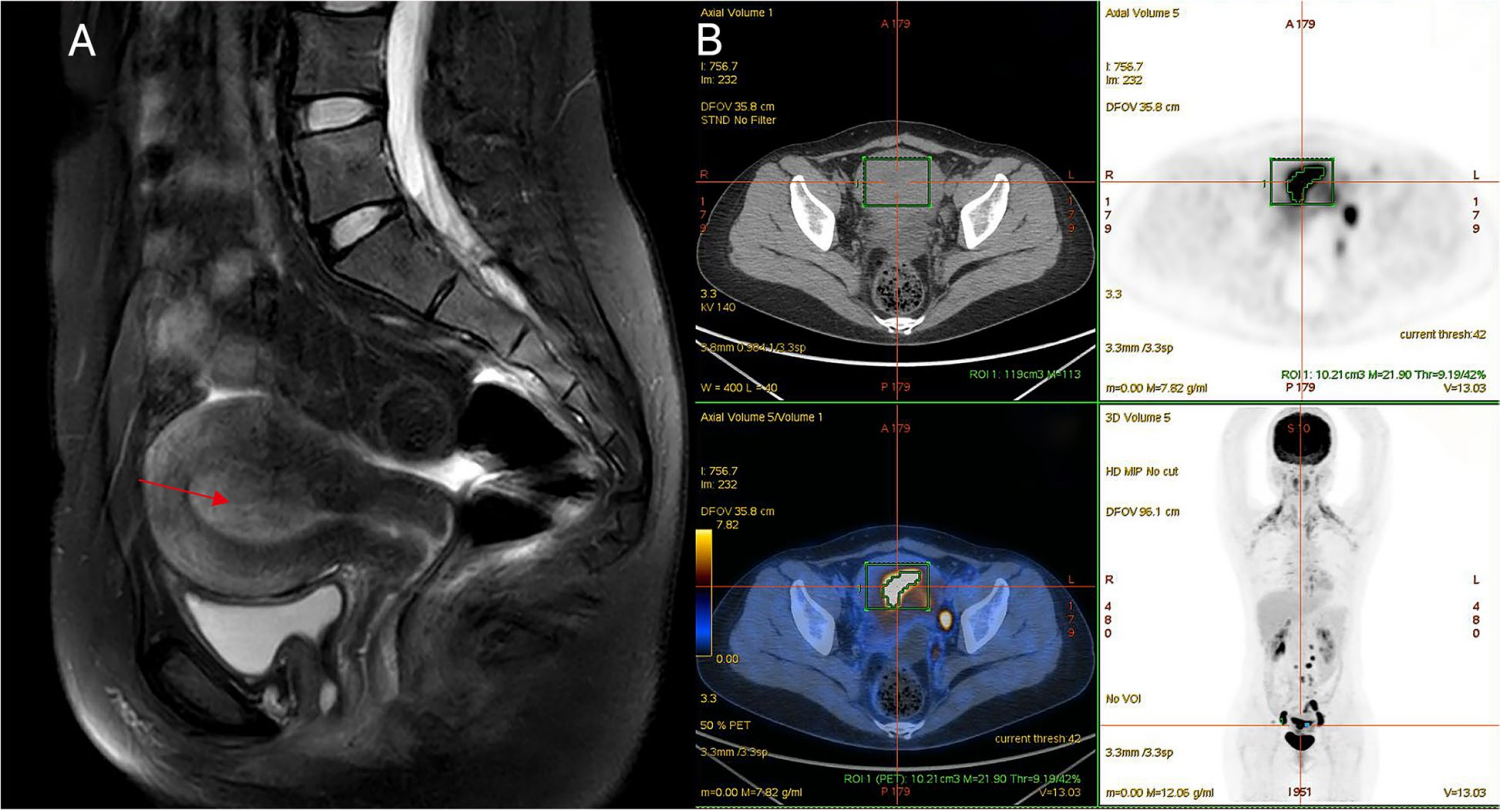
Imaging results of endometrial cancer. Magnetic resonance imaging shows that the endometrium is thickened, with a thickness of about 1.6 cm (red arrow), and the signal and enhancement are not uniform (A). Positron emission tomography/computed tomography shows increased fluorodeoxyglucose metabolism (SUVmax = 6.89) in the uterine cavity, consistent with endometrial cancer changes (B)
